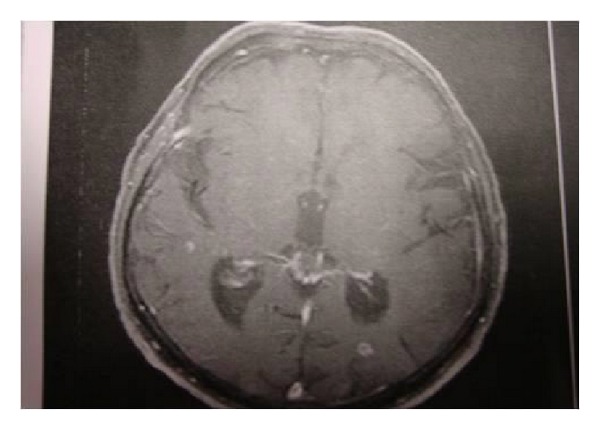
Axial MRI of the brain showing diffuse lesions mimicking secondary tumours.
